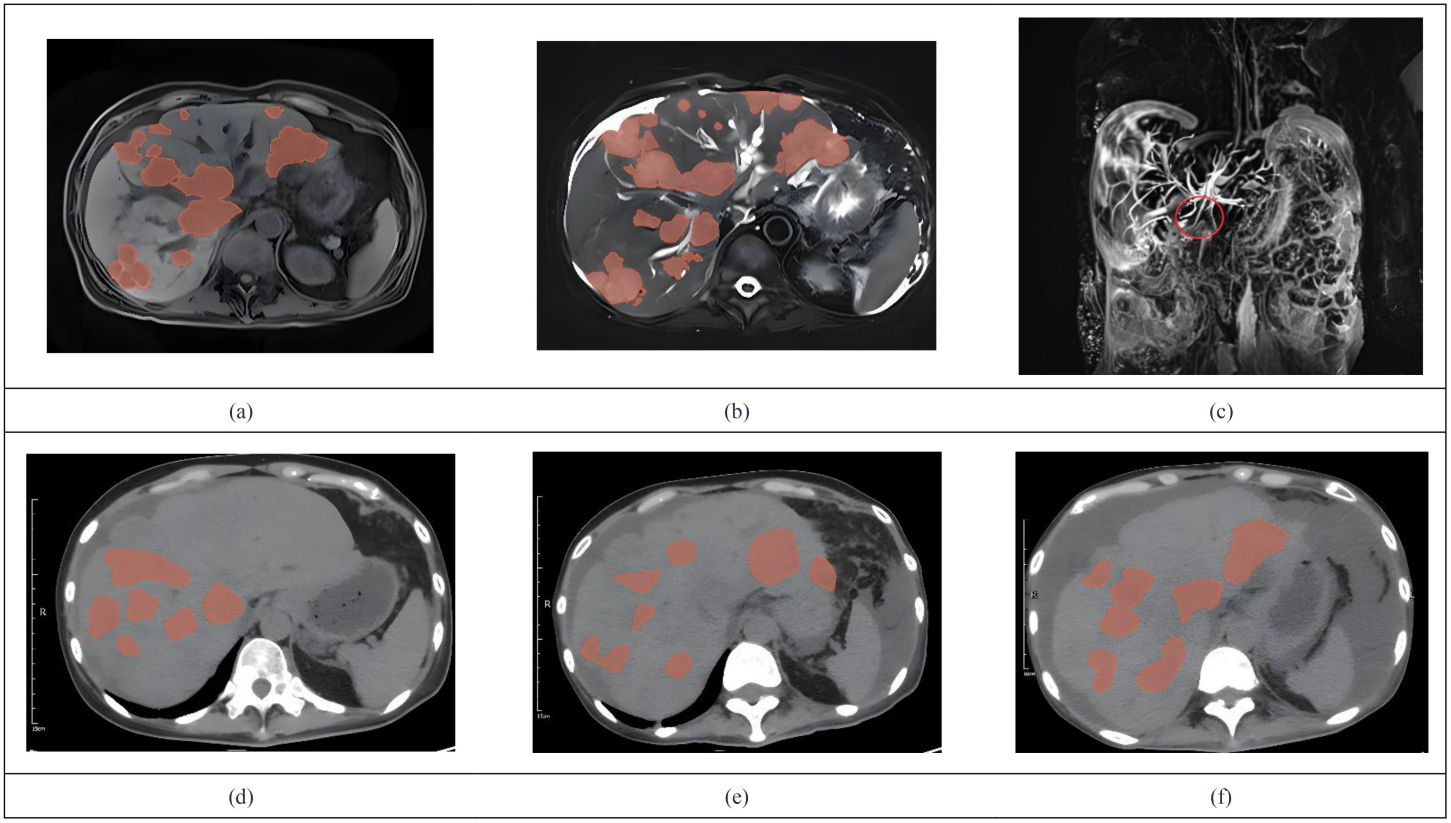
We used 3D Slicer (Version 5.9.0) to manually delineate the lesions by employing the “Draw” or “Paint” tools within the “Segment Editor” module. MRCP showed tumor invasion (a) Metastases demonstrate hypointensity on T1WI. (b) Metastases demonstrate hyperintensity on T2WI. (c) The coronal MRCP image showed a biliary duct interruption at the marked site. (d) The abdominal CT in July showed multiple low-density lesions in the liver. (e) Abdominal CT in august revealed progression of multiple low-density liver lesions both in number and size. (f) Abdominal CT in October showed no significant progression of tumor lesions.
